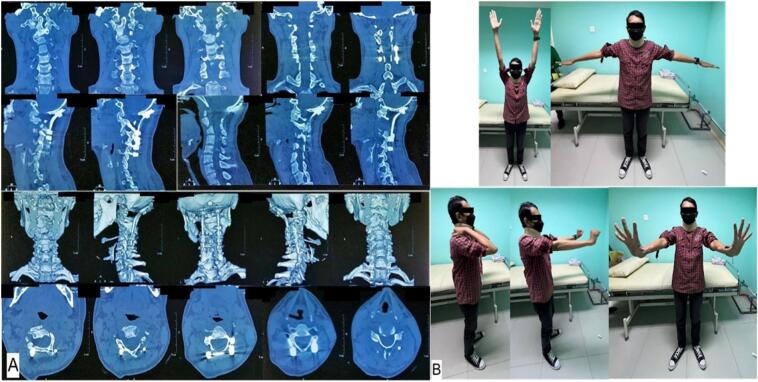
(A) Post-operative cervical CT-scan; (B) Post-operative range of motion shows improvement Frankel scale was observed 4 months post-operative.
